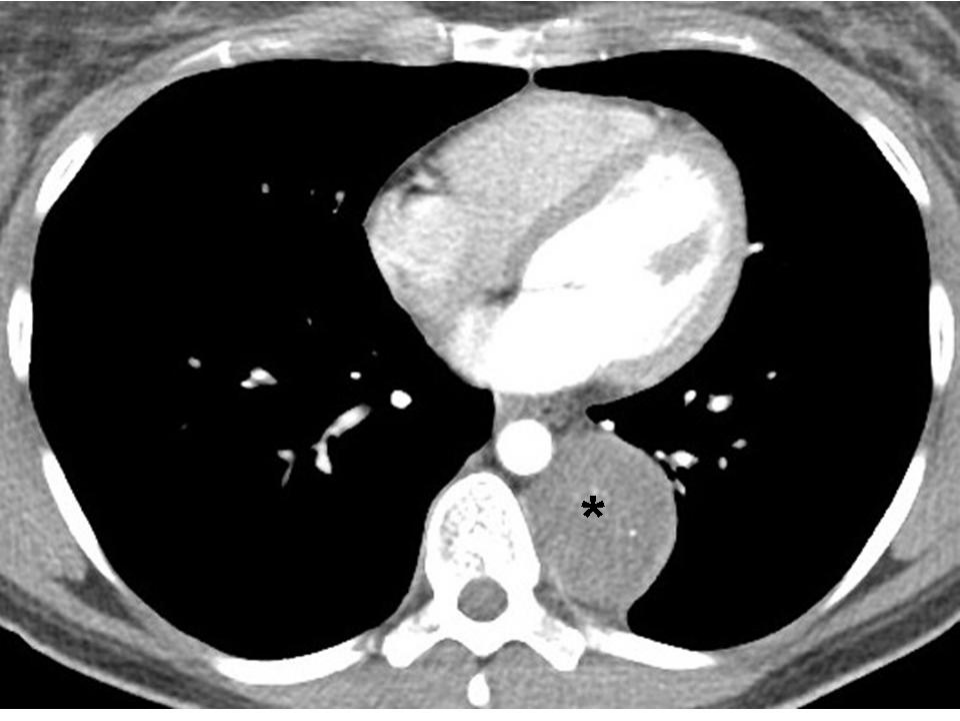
Contrast enhanced CT of a 35 years old female with history of vague chest pain for few months shows a well marginated enhancing mass (asterisk) in posteromedial aspect of the left hemi-thorax in para-vertebral location. The mass forms an obtuse angle with the chest wall suggesting that it is most likely extra-pulmonary in location. Main differential diagnosis of a solid mass in this location is a neurogenic tumor. These tumors can arise either from nerve roots (schwanoma, neurofibroma etc.) or sympathetic ganglia (neuroblastoma, ganglioneuroma, ganglioneuroblastoma etc.)
